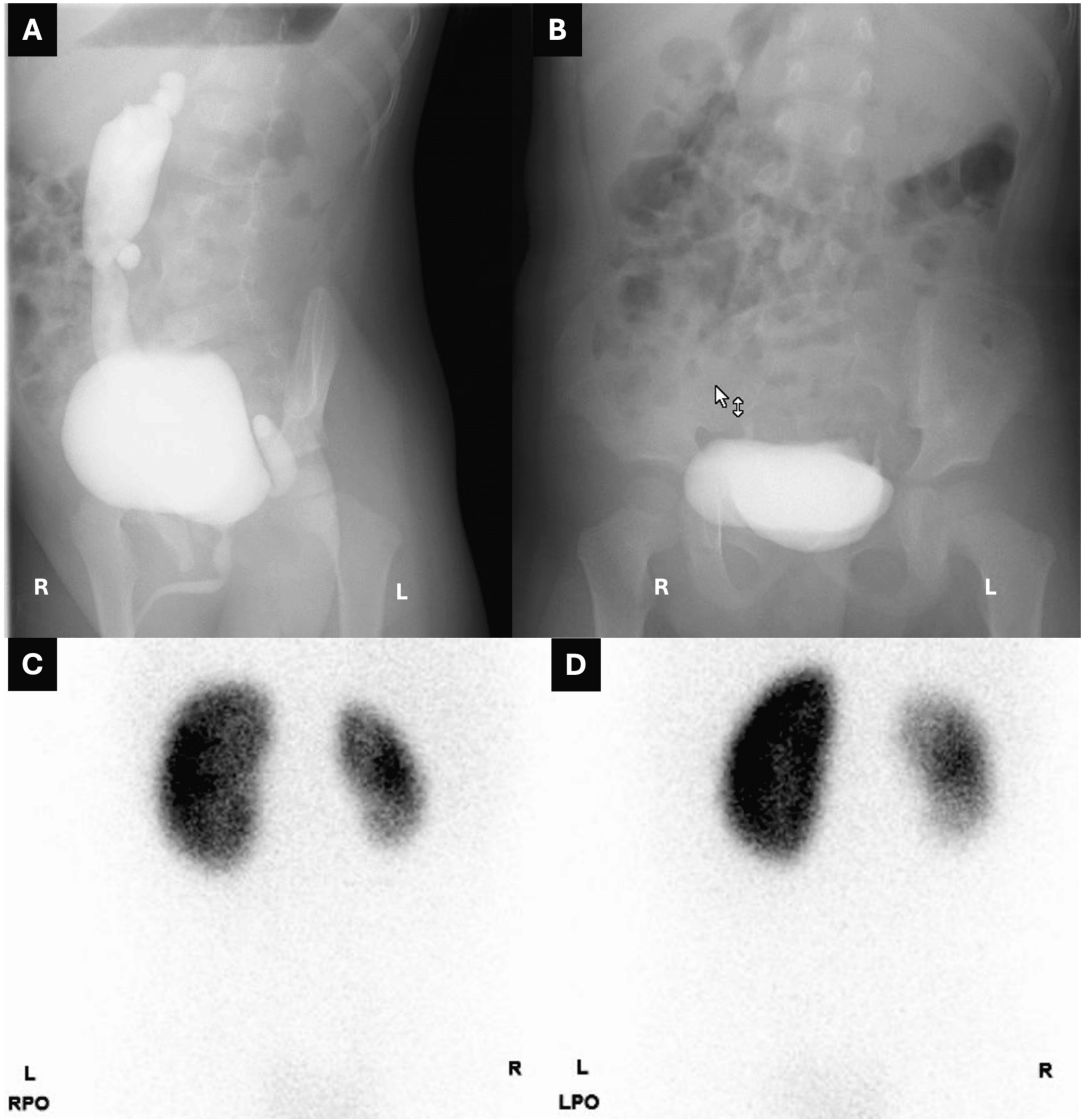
Voiding cystourethrography (VCUG) and renal scintigraphy images The VCUG disclosed grade 4 right and grade 1 left VUR. However, we should have noticed that the left side seems to be atypical, as grade 1 VUR retrospectively, bilateral ureteroneocystostomy had been scheduled. A: Voiding phase; B: Post-voiding image of frontal view 99mTc-dimercaptosuccinic acid (DMSA) static renal scintigraphy showed a right atrophic kidney and scarring. C: Right posterior oblique (RPO); D: Left posterior oblique (LPO)
